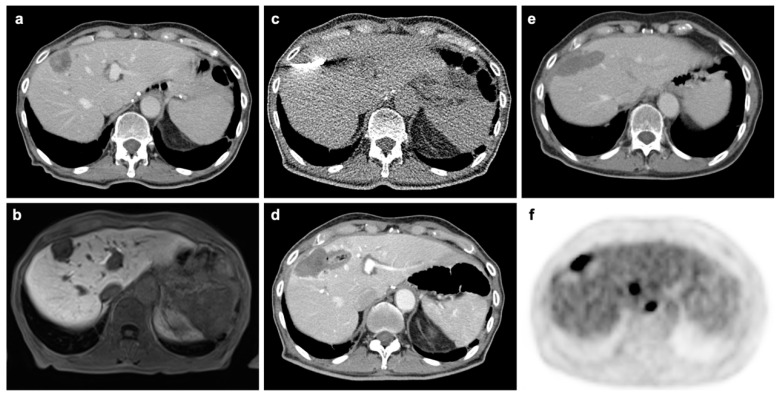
Patient from our institution undergoing microwave ablation (MWA) for oligometastatic liver disease. (a). Pre-interventional CT in portal-venous phase shows contrast-enhancing metastasis in segment VIII. (b). Pre-interventional MRI in hepatocyte-specific phase. (c). Interventional imaging during MWA. (d). CT in portal-venous phase acquired immediately after intervention without evidence of complication or residual tumor. (e). Four-month follow-up F-18 FDG PET/CT without morphological evidence of recurrence in CT component. (f). PET-component acquired at same scan 4-month after MWA with tracer accumulation at the margins of the previous ablation in line with recurrence. Additional metastases without morphological evidence in the CT component can also be depicted.
